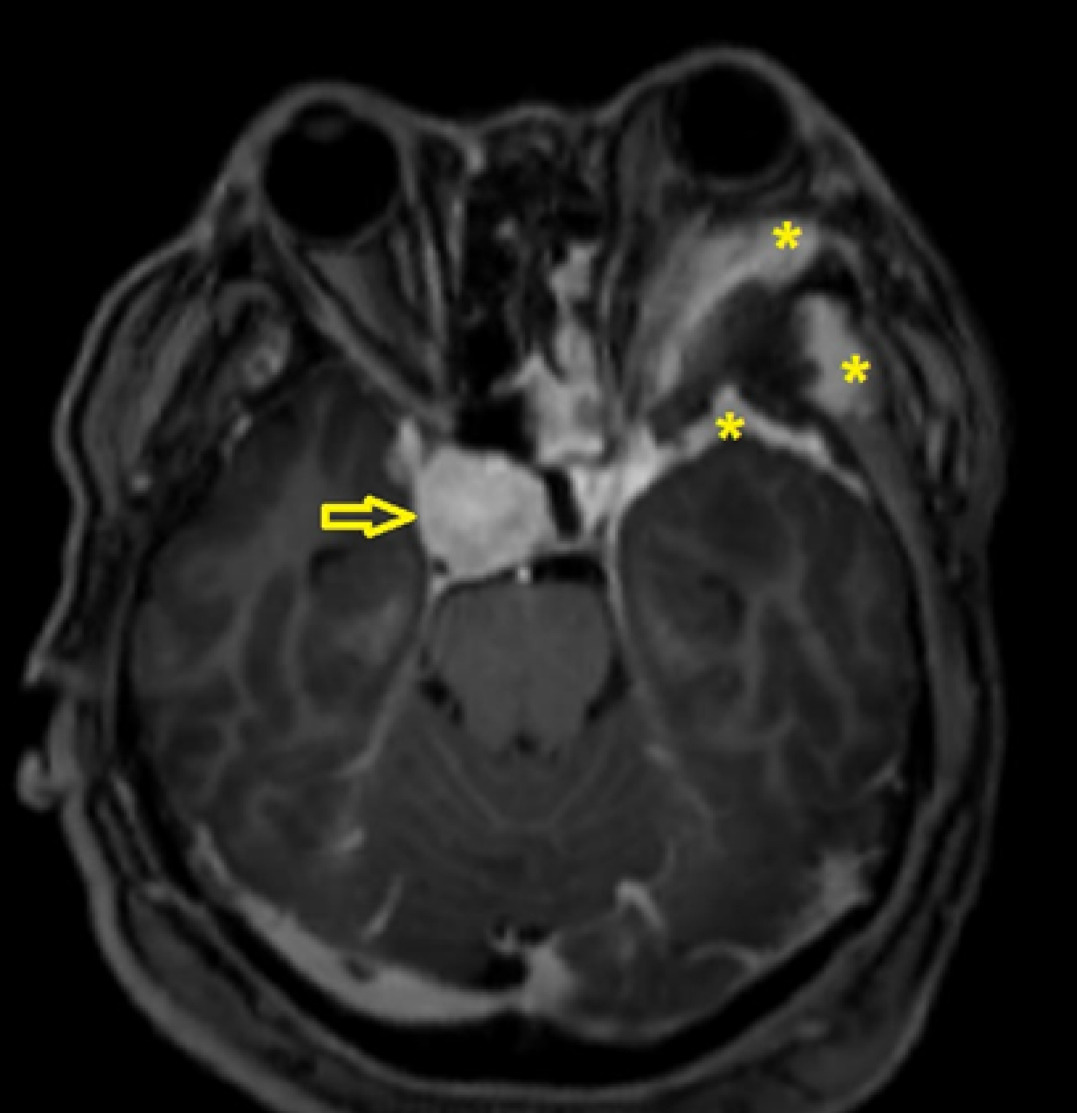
MRI with gadolinium showing enhancing soft tissue surrounding the lesion (*). Left orbital content is being pushed anteriorly. There is also presence of another lesion at parasellar region which showed vivid enhancement (open arrow). Both lesions are not in continuity with one another.
